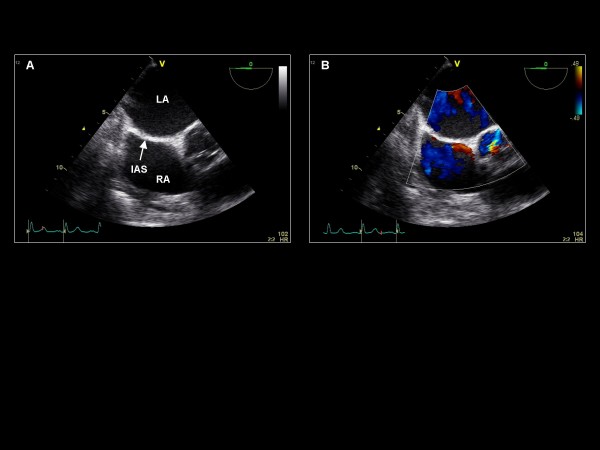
Further withdrawal and anterior rotation of the probe at imaging plane 0° reveals the (A) The interatrial septum (IAS). (B) Color Doppler demonstrates no flow across the IAS.
